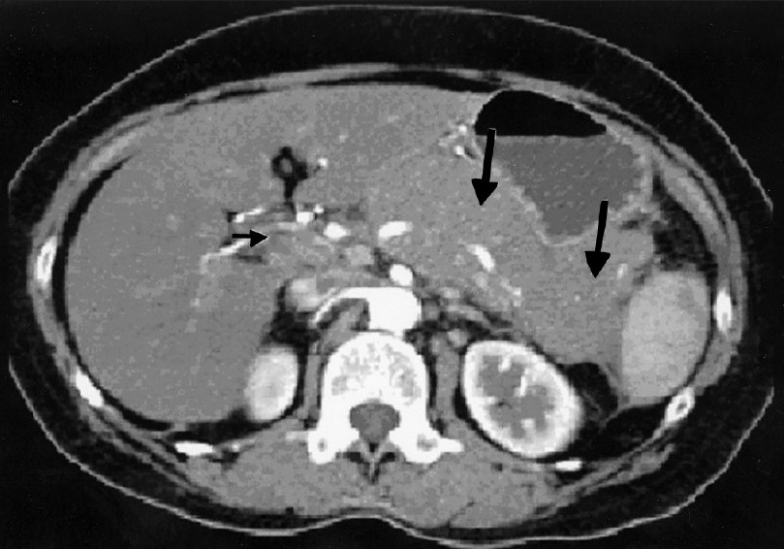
CT scan showing diffuse homogenous enlargement of pancreatic parenchyma (large arrow) with regional nodal disease (small arrow)
